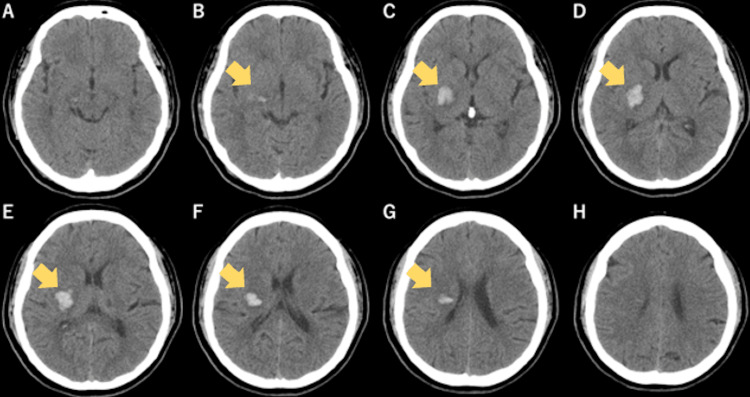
Initial CT imaging revealed a cerebral hemorrhage extending from the right putamen to the thalamus In the initial head CT scan immediately after onset, hemorrhage was observed in the right putamen, posterior limb of the internal capsule, and thalamus (B-G).
